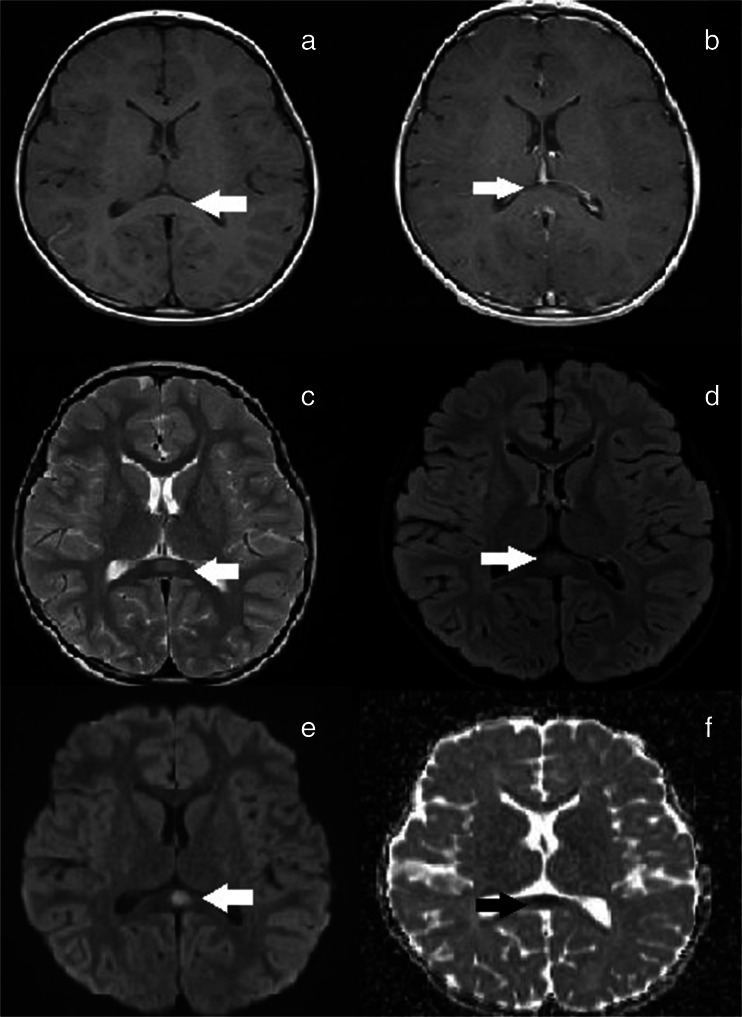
The hyperintense region in T2-weighted and fluid-attenuated inversion-recovery (FLAIR) MRI, a well-defined lesion in the splenium of the corpus callosum with restricted diffusion in diffusion-weighted MRI (DWI) and no enhancement in post contrast T1-weighted (MRI Axial T1W precontrast (a) postcontrast (b) no lesion enhancement; Axial T2W (c), FLAIR (d), DWI (e) lesion marked using white arrow , ADC (f) - black arrow)
